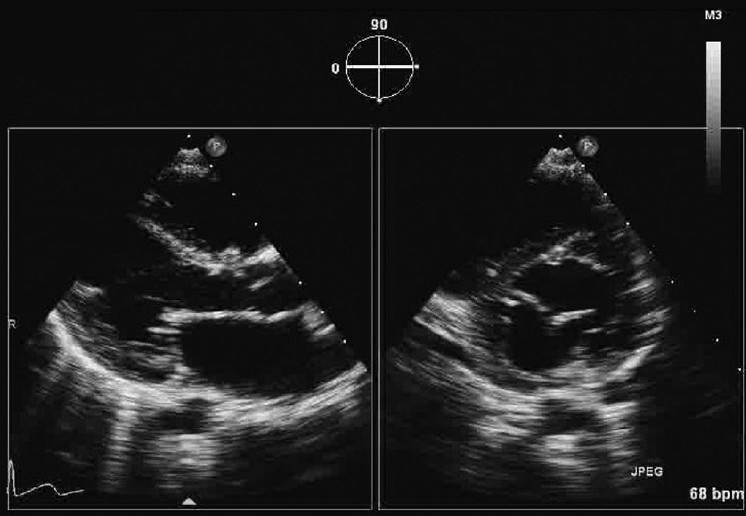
Simultaneous parasternal long axis and short axis view showing two separate mitral orifices
